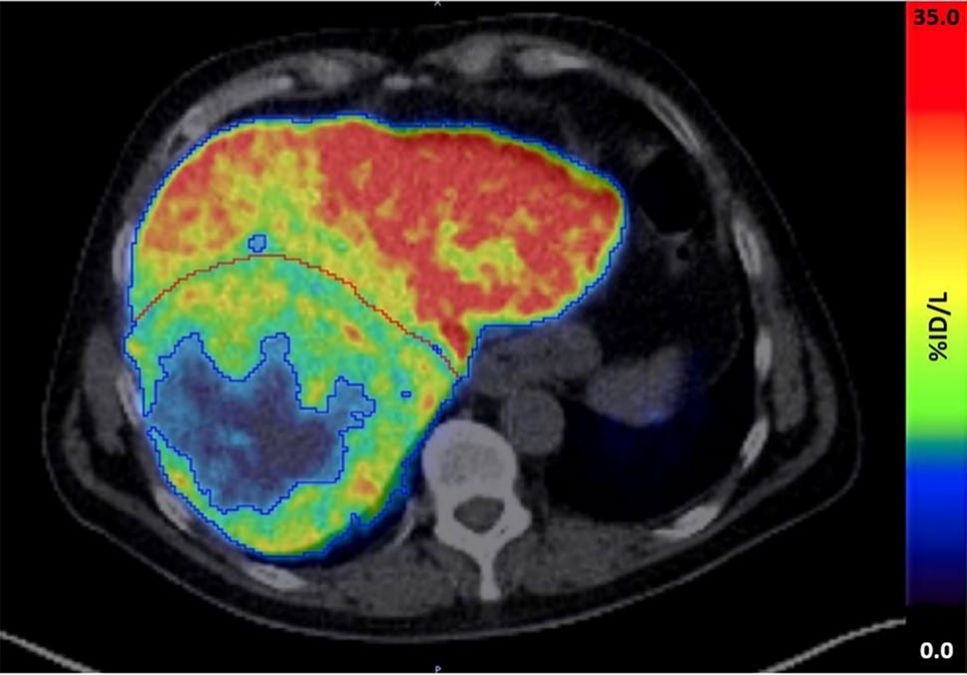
Fused axial abdominal ^18^F-FDGal PET/CT scan of a patient with a large HCC with central part with low metabolic activity. Blue line: whole liver VOI with a tissue threshold of 6 kBq/m. Red line: tumor boundary. The tumor area with low metabolic activity is included in Table 2
